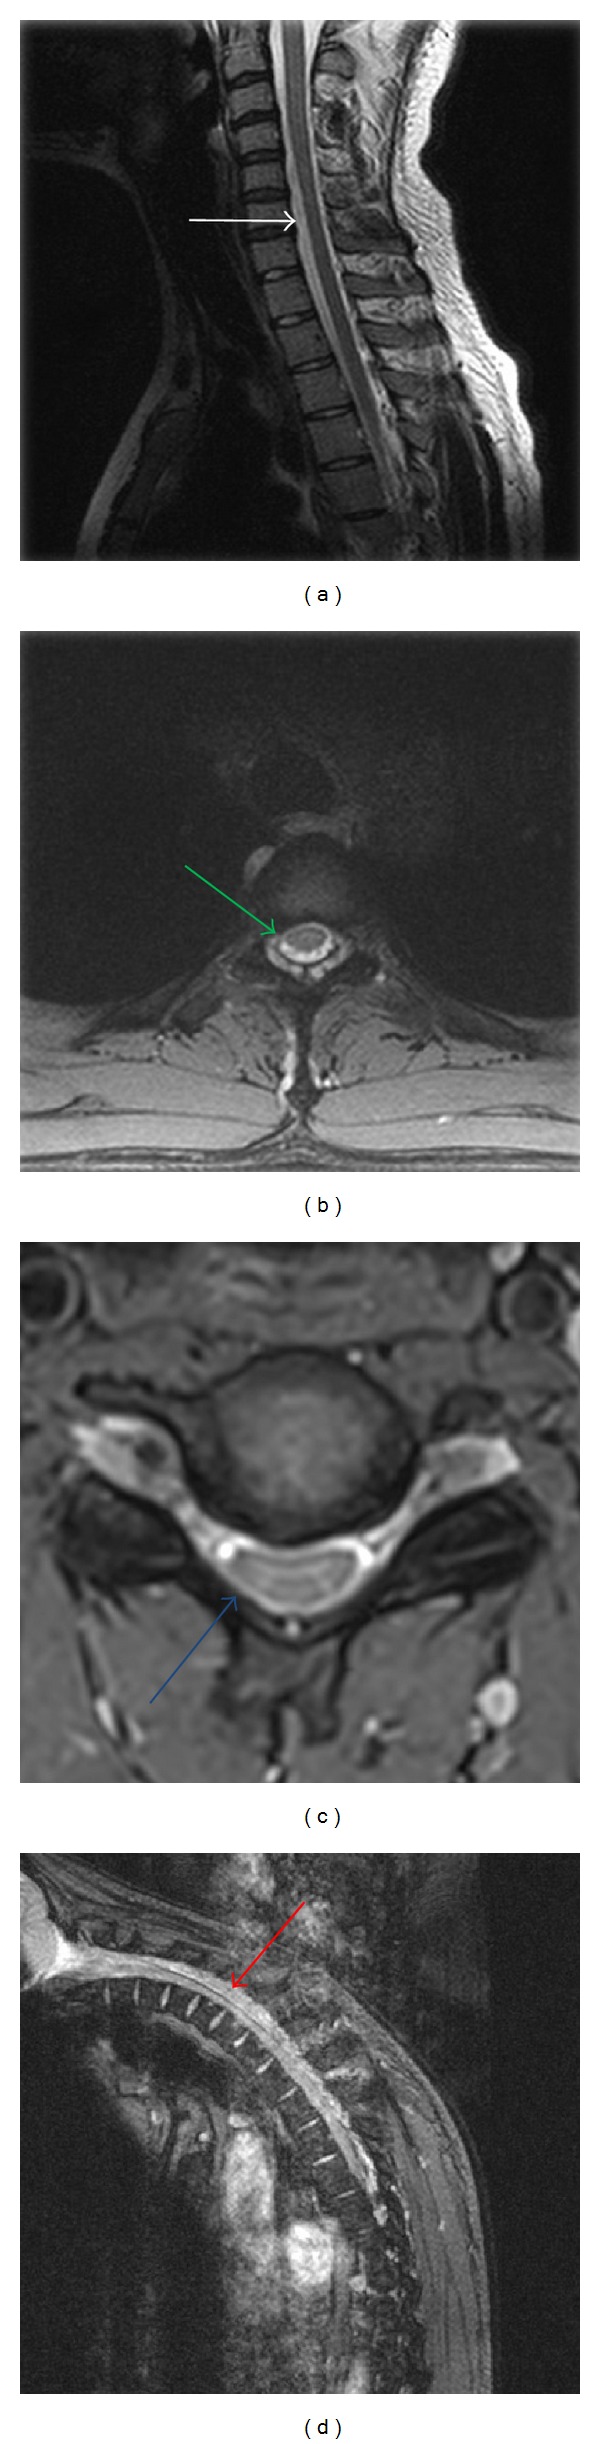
Localised cord atrophy (white arrow) on neutral sagittal T2WI (a), pear shaped asymmetric cord flattening on neutral transverse T2WI (green arrow) (b), asymmetric cord flattening with loss of attachment of dura from subjacent lamina (blue arrow) on neutral position axial T2WI MRI (c), and anterior displacement of posterior dura from C3–D8 on postcontrast imaging showing intense enhancement of posterior epidural space from C6–D3 (red arrow) (d).
